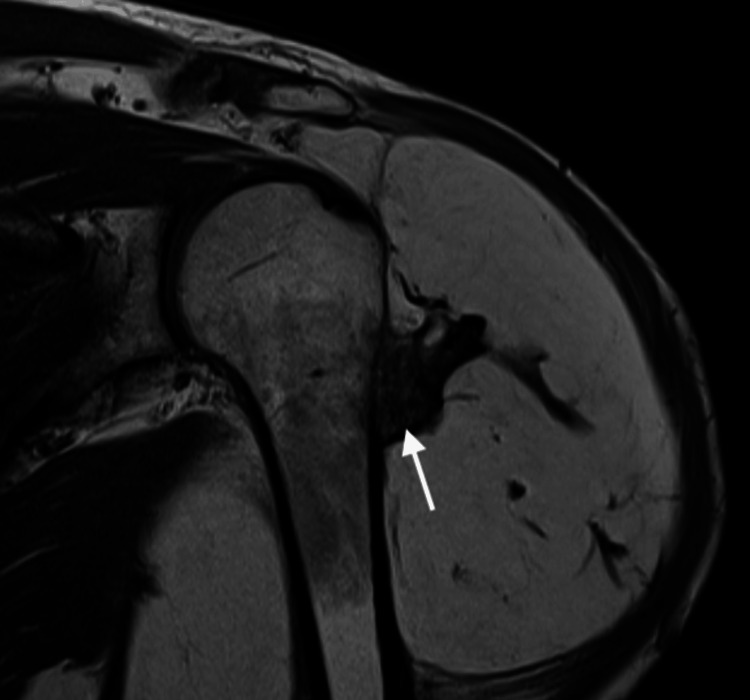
Coronal T1-weighted MRI. A hyperintense lesion adjacent to the left humerus, consistent with fatty tissue, can be observed. The lesion includes a bony prominence adjacent to the cortical surface (arrow), with no apparent continuity with the humeral bone marrow.
